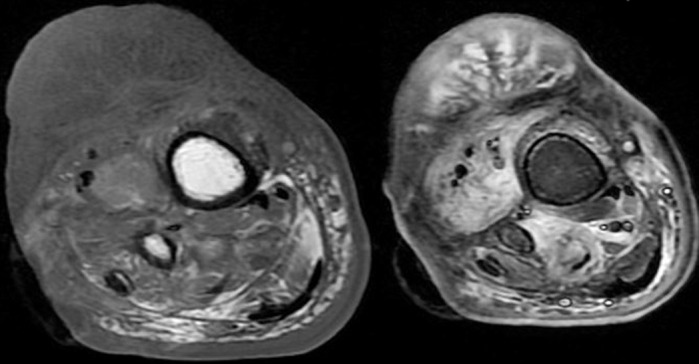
MRI of the same patient (axial T1 and T2 STIR images) is consistent with the CT findings and shows no bone marrow involvement
